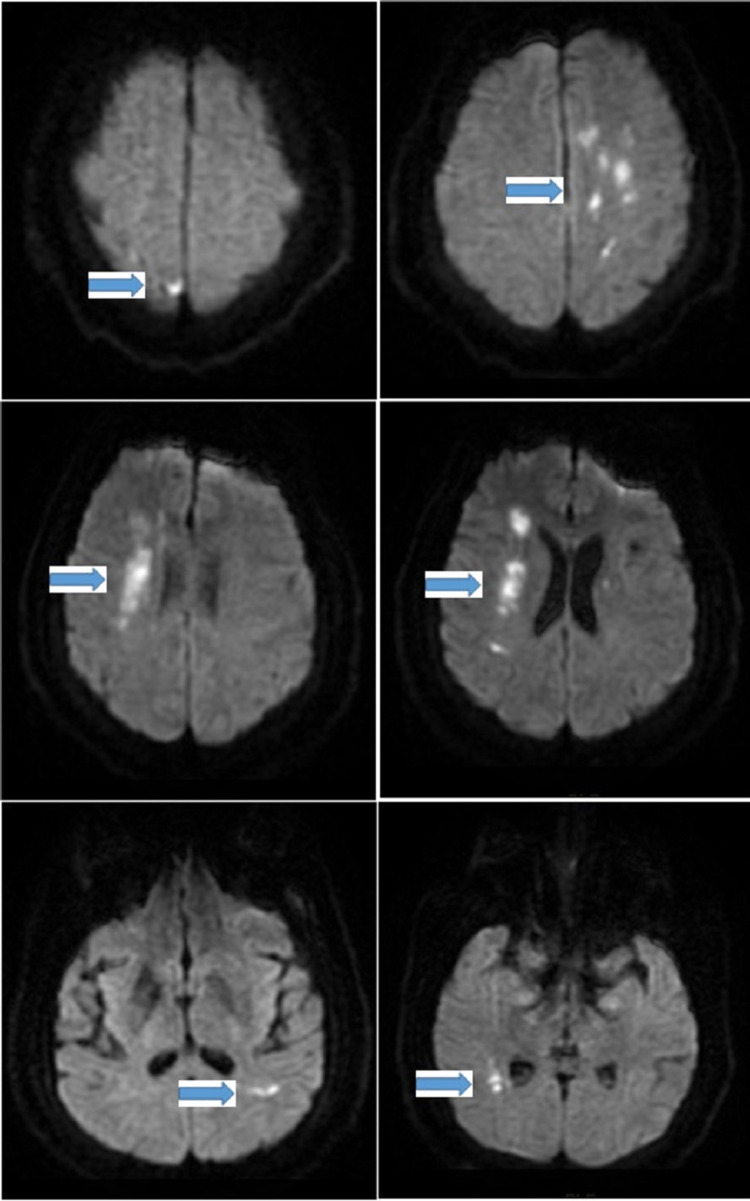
MRI brain showing multiple bilateral patchy small areas of acute infarctions related to watershed distribution (arrows) MRI: magnetic resonance imaging
